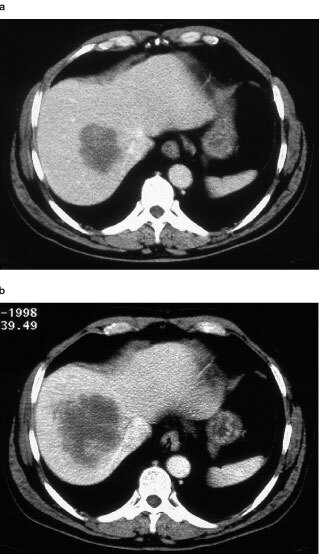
A 55-year-old patient with colorectal metastases to the liver. (a) Pretreatment CT scan in segment 8 (portal venous phase). (b) CT scan (portal venous phase) at 8 weeks after the pretreatment evaluation and 2 weeks after the fourth treatment. Increased tumour necrosis is evident after treatment.
